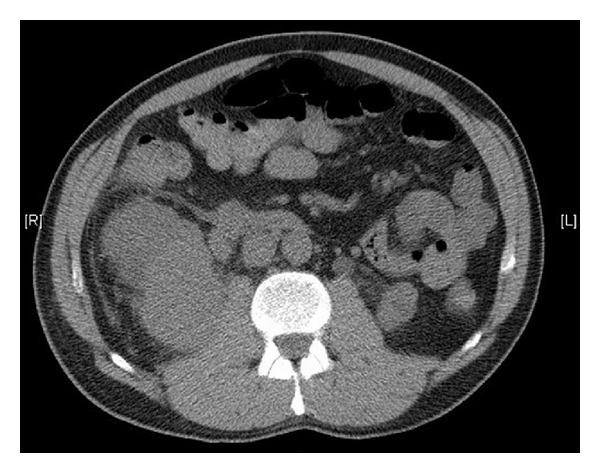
Axial CT image demonstrating primary plasmacytoma of the kidney.
